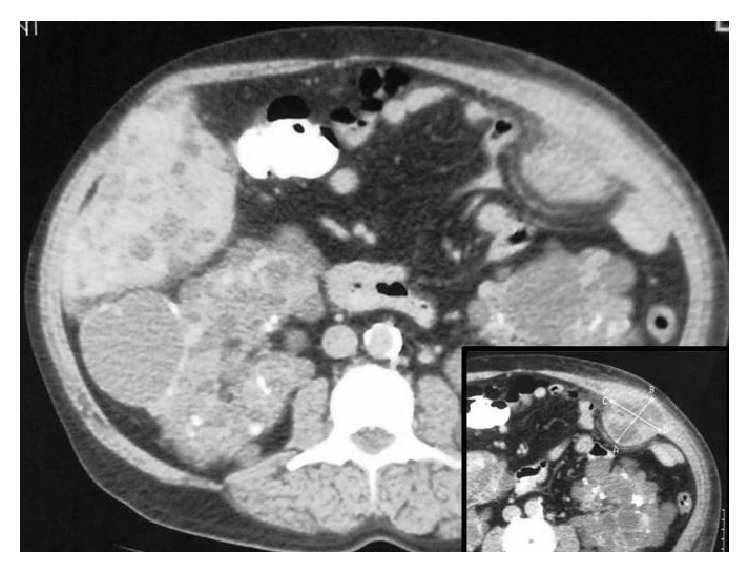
Abdominal Computed Tomography: abscess of the left anterior abdominal wall.
